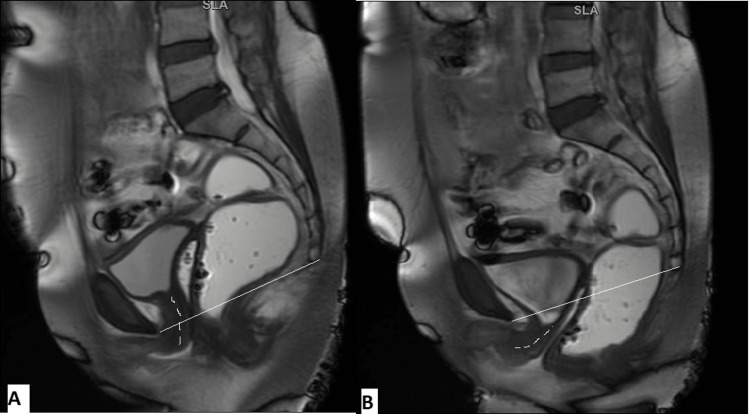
A 45-year-old female complaining of urinary symptoms. (A) Midline sagittal dynamic FIESTA MRI at rest reveals the normal position of the urethra. (B) In sagittal dynamic FIESTA MRI in the maximal strain phase, the urethra is seen inferior to the pubic symphysis in horizontal orientation. Dotted line: urethra; solid line: pubococcygeal line; FIESTA: fast imaging employing steady-state acquisition.
